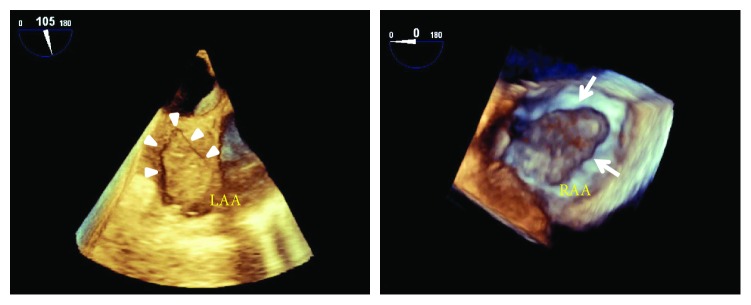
Three-dimensional transesophageal echocardiographic images of the left (arrow heads) and right (arrows) atrial appendage thrombi. Abbreviations are the same as in Figure 2.
